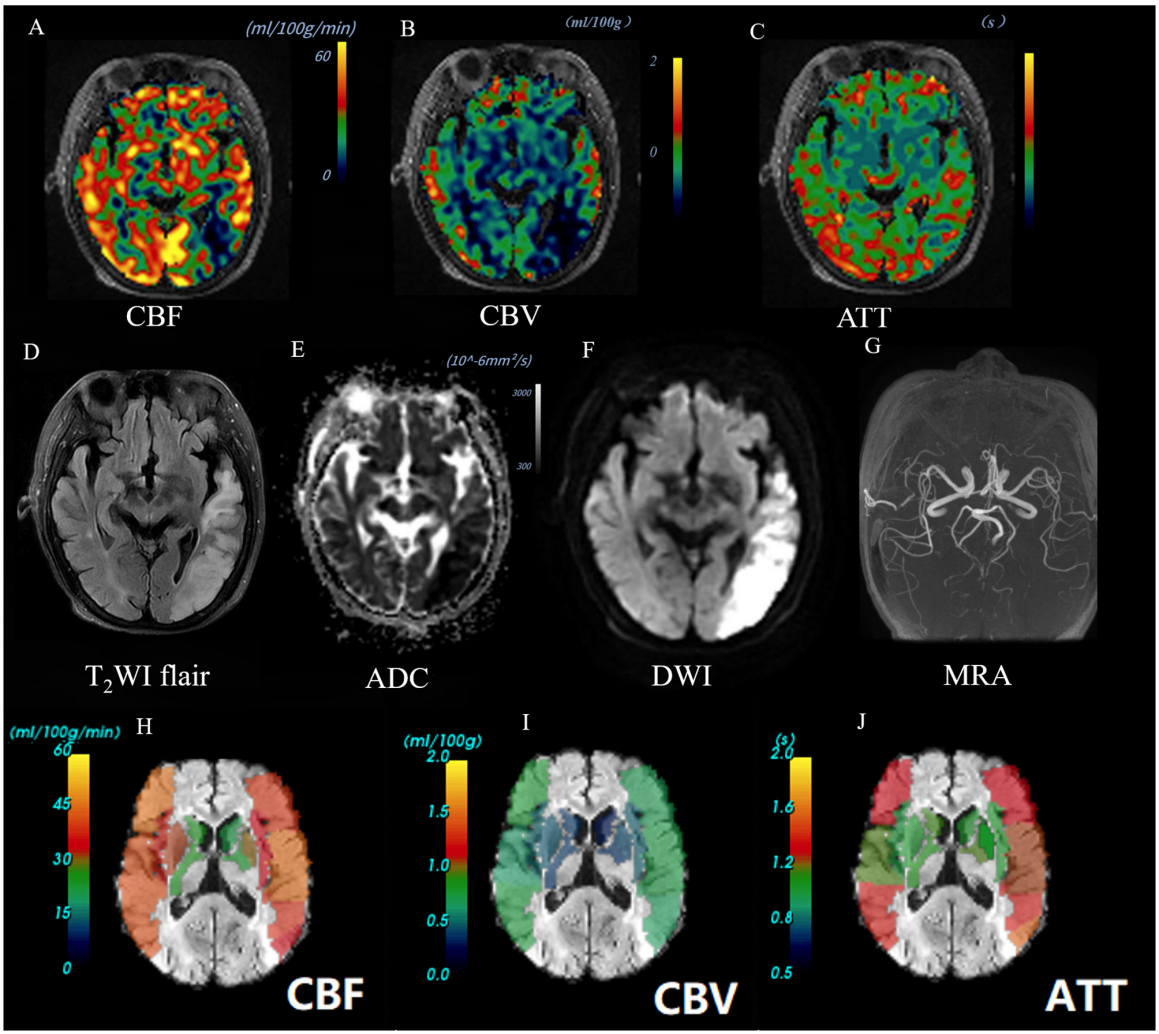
Images of an AIS patient with a good outcome (90-day mRS = 0). A 66-year-old female patient was diagnosed with AIS in the left temporal lobe. Baseline NIHSS score of 1 point, 90-day mRS score of 0 point. The CBF perfusion map (A) and CBV perfusion map (B) showed low perfusion of the lesion. The ATT perfusion map (C) revealed an insignificant change. The lesion showed high signal on T_2_Flair (D) and DWI map (b = 1,000) (F), and the ADC value decreased (E). The 3D-TOF-MRA map showed no obvious stenosis or occlusion (G). The ASPECTS template was used to standardize the perfusion parameters across 10 different zones. (H–J) The scores obtained were as follows: CBF-ASPECTS: 8 points, CBV ASPECTS: 9 points, ATT-ASPECTS: 10 points. Warm-to-cold color bars represent values from high to low.
